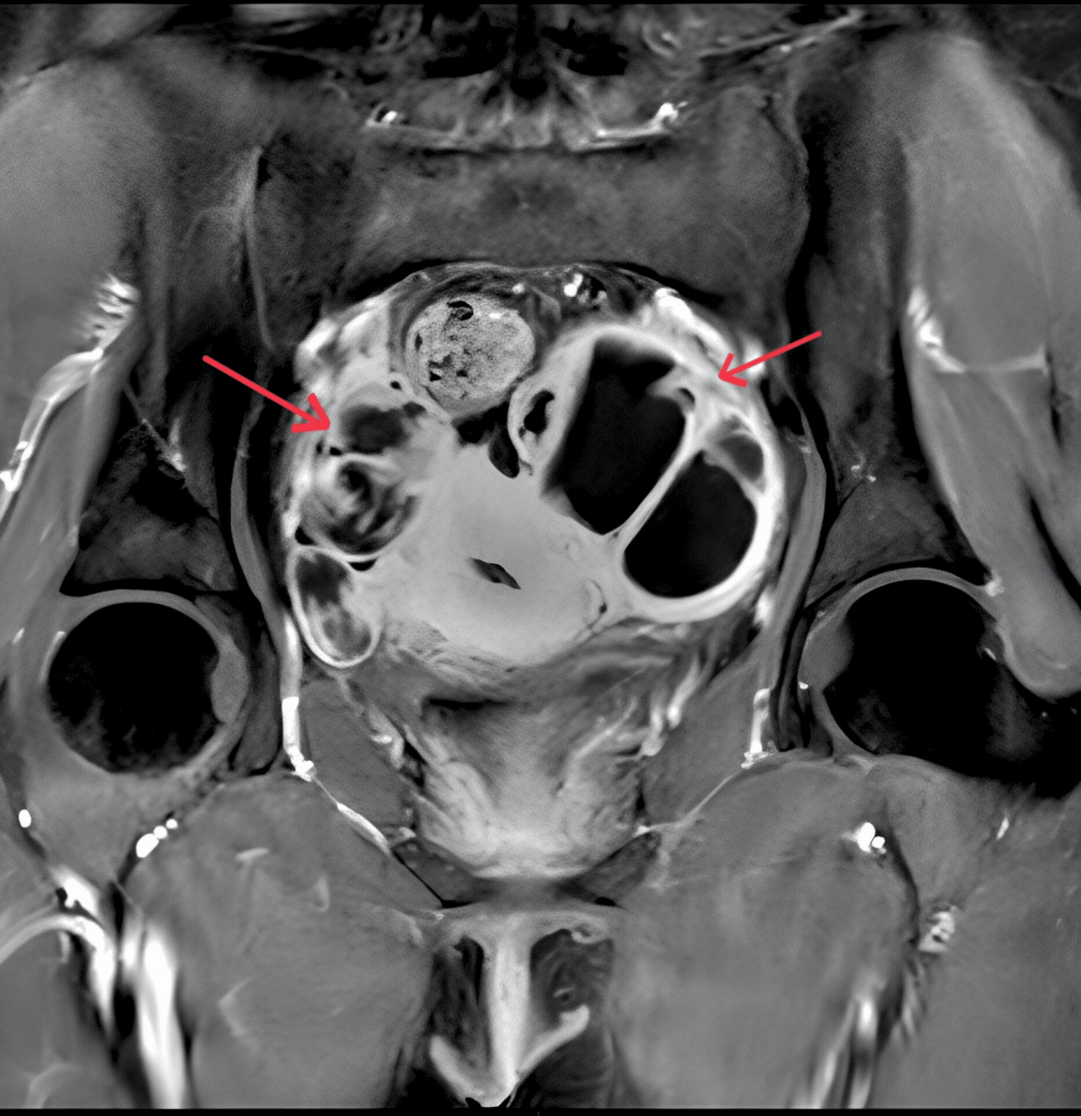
A contrast-enhanced coronal MRI shows a peripherally enhancing, multiloculated collection in the bilateral adnexa, consistent with tubo-ovarian abscesses (arrows).
